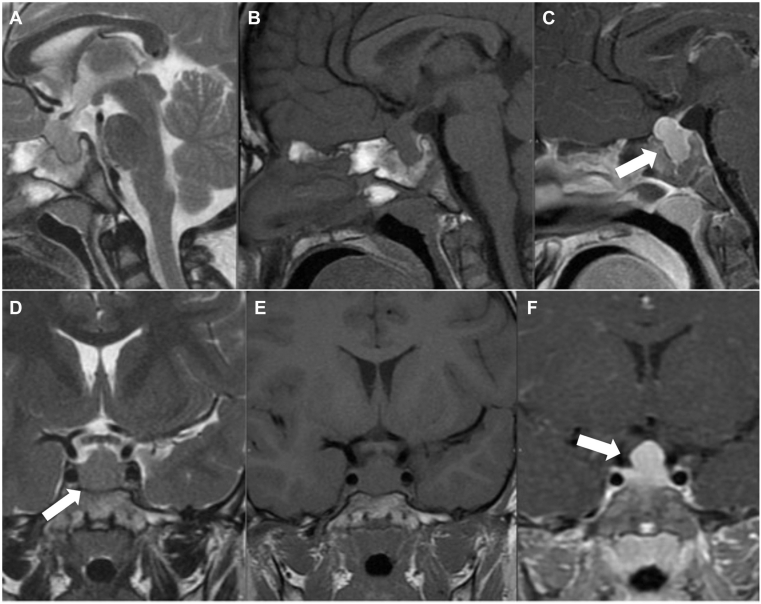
Initial pituitary MRI. (A–C) Sagittal T2, T1, and T1 postcontrast sequences show diffuse homogeneous enhancement of an isointense sellar lesion (white arrows) with mild upward displacement of the optic chiasm. (D–F) Coronal T2, T1, and T1 postcontrast dynamic sequences demonstrate a centrally located pituitary stalk with contact of the optic chiasm (white arrows). MRI = magnetic resonance imaging.
